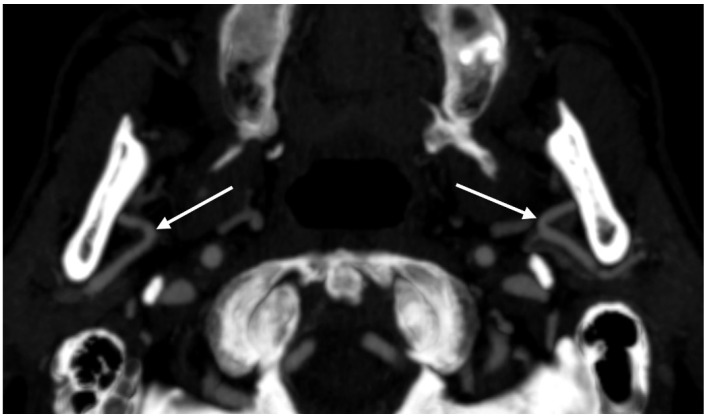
Axial CTA slice viewed inferiorly. Bilateral medial loops (arrows) of the maxillary arteries.
